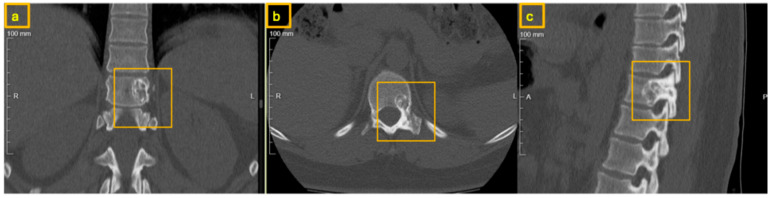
CT of the thoracic spine after the percutaneous biopsy. The yellow square highlights the extent of the nidus within the posterior column, involving the root of the left T11 pedicle, (a) coronal view, (b) axial view, and (c) sagittal view.
